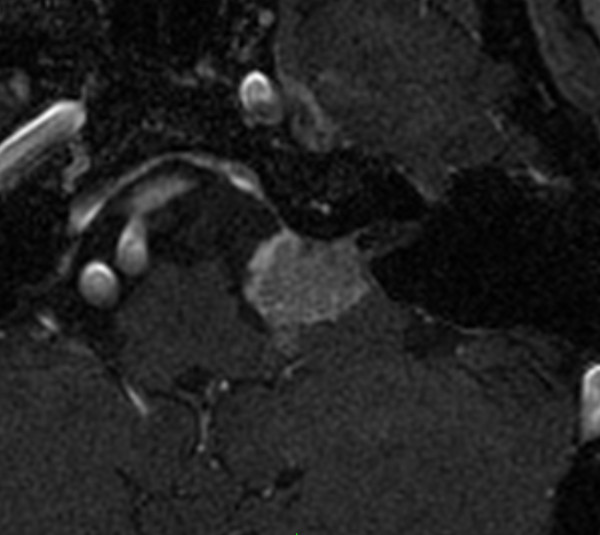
Pre-treatment MRI. Enhanced T1-axial MRI of the brain prior to gamma knife radiosurgery showing an enhancing lesion extending into the cerebellopontine angle from the left internal acoustic meatus.
